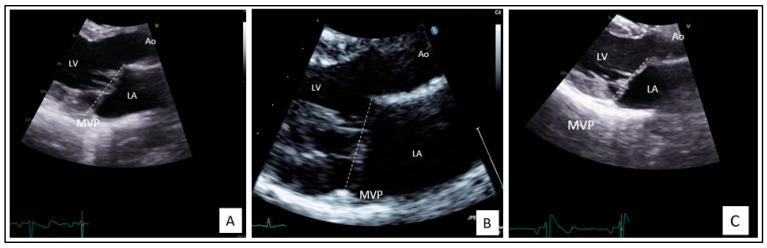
Echocardiography of the index case (A), the affected daughter (II-2) (B), and the oldest daughter with a prodromal MVP (II-1) (C). LV: left ventricle; LA: left atrium; Ao: aorta; MVP: mitral valve prolapse.
